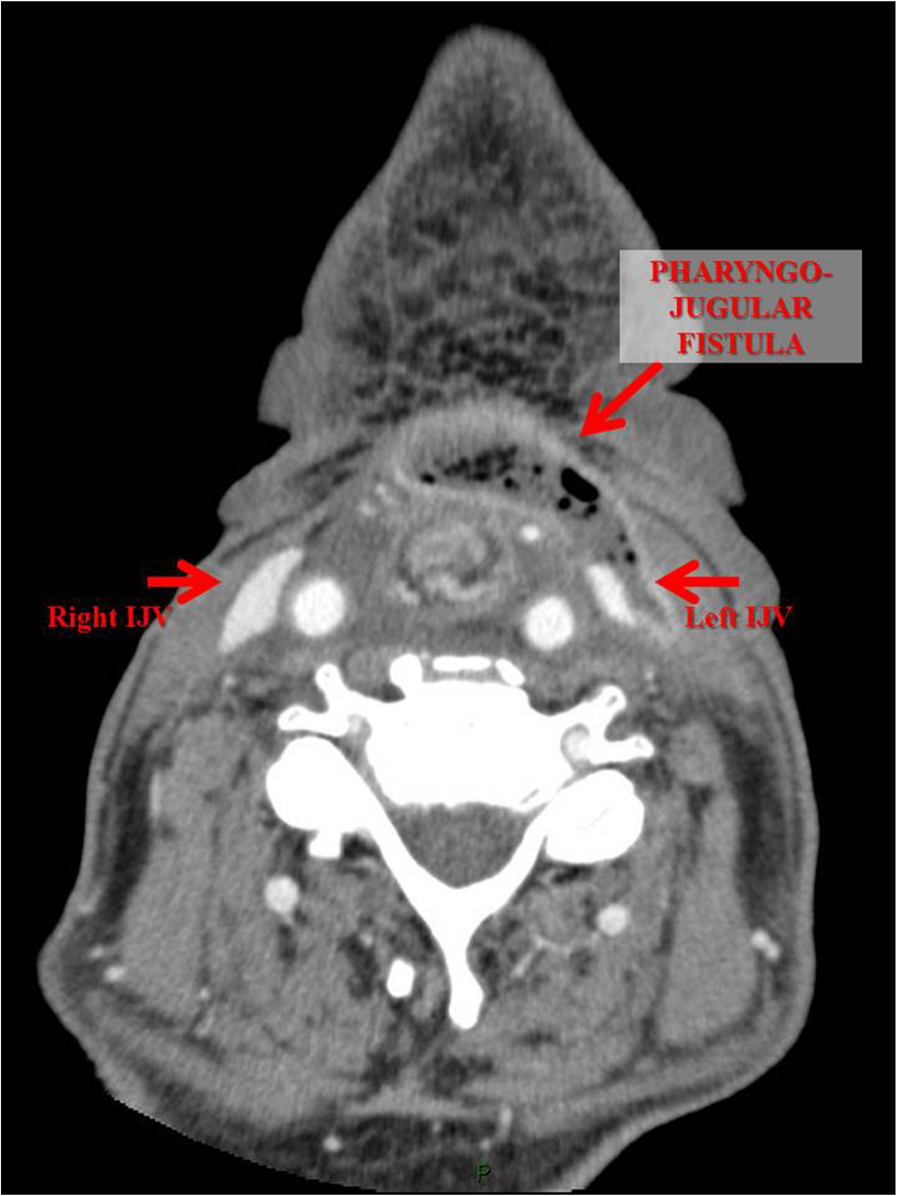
Neck computed tomography (axial view) showed the presence of a fistula between the left internal jugular vein and pharynx
